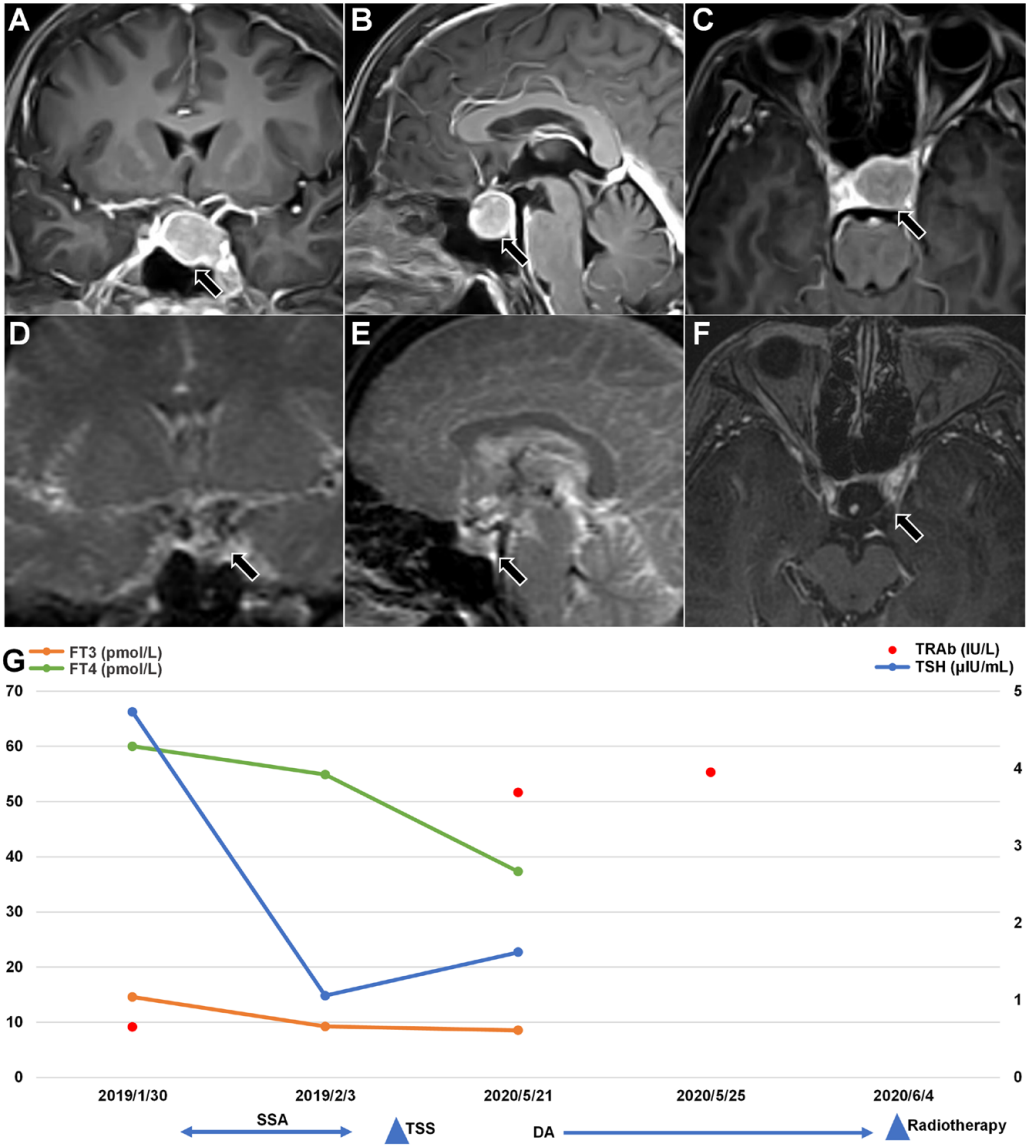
Pituitary-enhanced MRI, thyroid function change, and treatment of case 3. DA, dopamine receptor agonist; FT3, free tri-iodothyronine; FT4, free thyroxine; SSA, somatostatin analog; TRAb, thyroid-stimulating hormone receptor antibodies; TSH, thyroid-stimulating hormone; TSS, transsphenoidal surgery. Normal ranges: FT4 7.64–16.03 pmol/L, FT3 3.28–6.47 pmol/L, TSH 0.49–4.91 μIU/mL, TRAb 0–1.7 IU/L.
